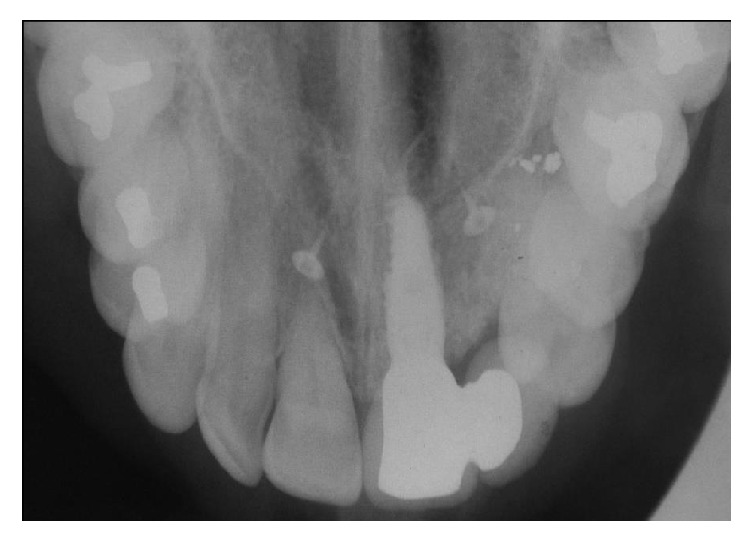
Standard occlusal radiograph taken at initial consultation. The orifices of bilateral nasopalatine canals can be seen clearly apical to the central incisor teeth—the incisive canals: one at the apex of the implant and the other at the apex of natural tooth 11.
